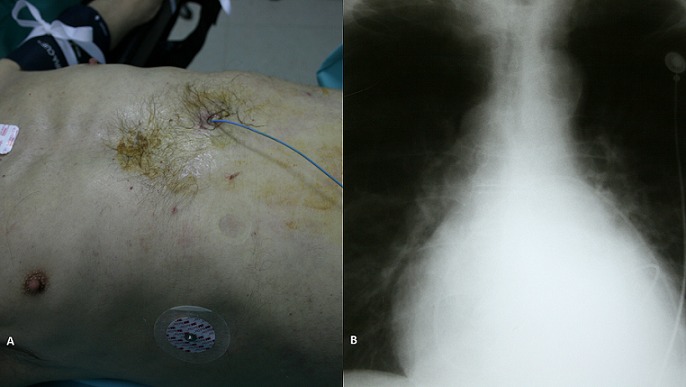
(A) pericardiocentisis with catheter insertion; (B) chest X-ray showing pericardial catheter winded to the pericardium
